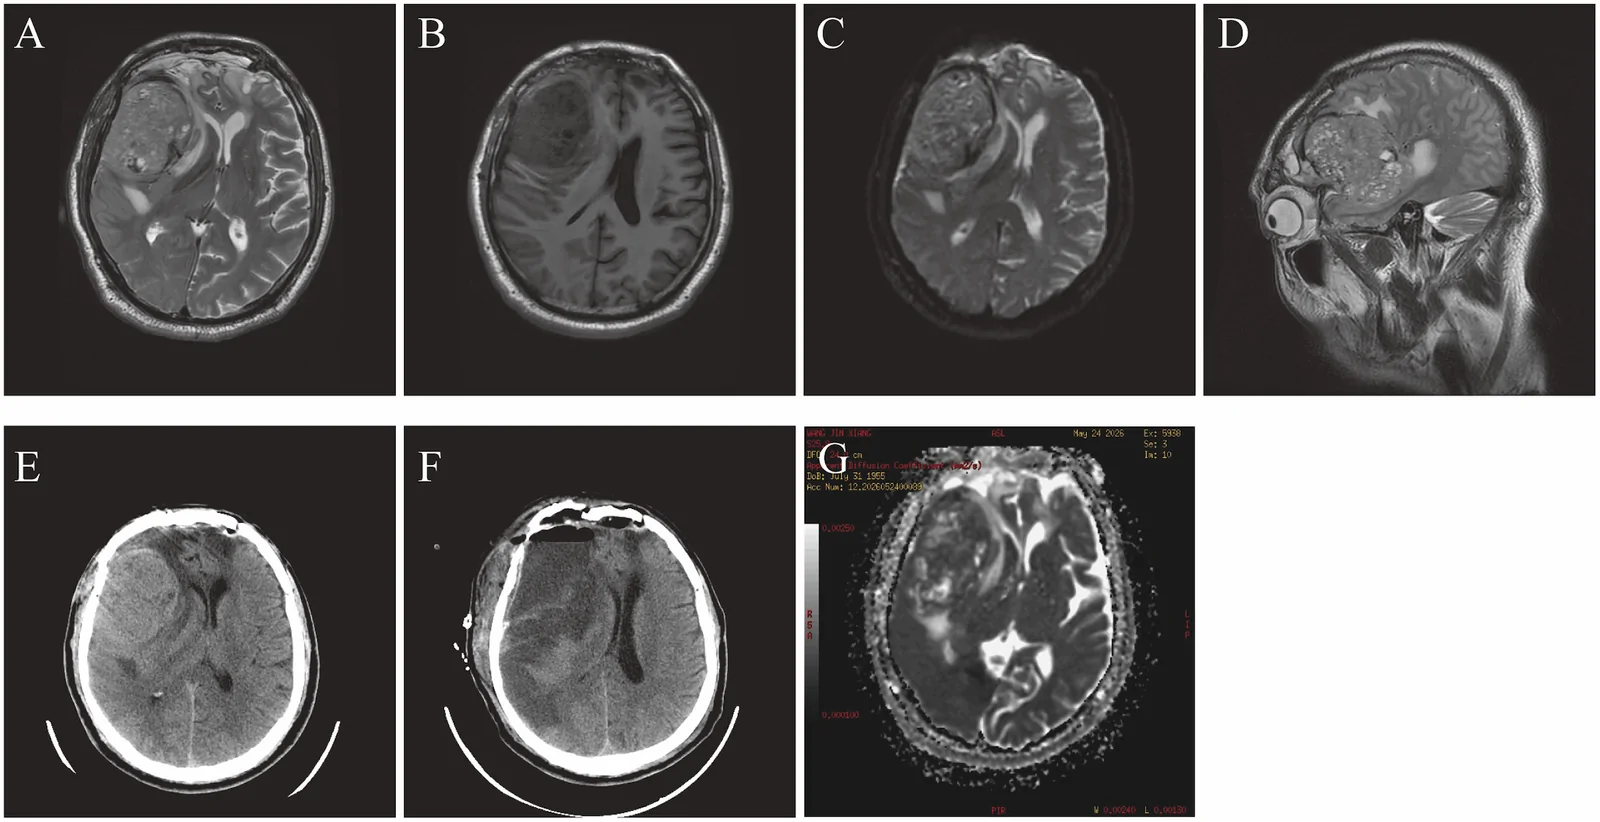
Imaging findings of recurrent intracranial SFT. (A) Axial T2-weighted MRI shows a mass in the right frontal-temporal lobe with extensive dural attachment, mass effect, ventricular compression, and leftward midline shift. (B) Contrast-enhanced T1-weighted MRI shows marked homogeneous enhancement. (C) Diffusion-weighted imaging (DWI) shows mixed signal intensity without obvious diffusion restriction. (D) Sagittal T2-weighted MRI shows the anteroposterior extent of the lesion. (E) Preoperative axial CT scan shows a high-density extraxial mass. (F) Postoperative axial CT scan confirms gross complete resection. (G) ADC map. The lesion was diagnosed as a recurrent meningioma based on imaging findings.
